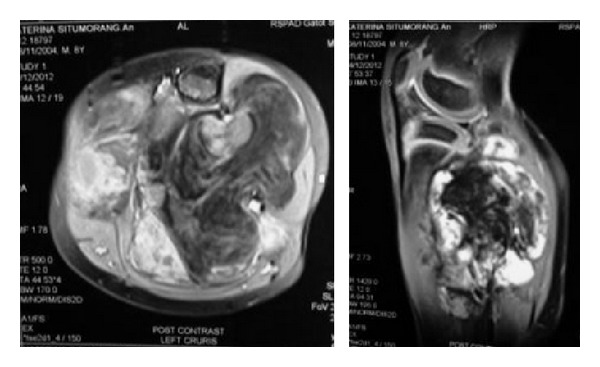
MRI of Case  3. Axial and sagittal view in T1-weighted images with fat saturation and contrast administration. The mass looked more infiltrative to surrounding muscles, with hypointense fatty component and mildly enhanced myxoid tissue.
